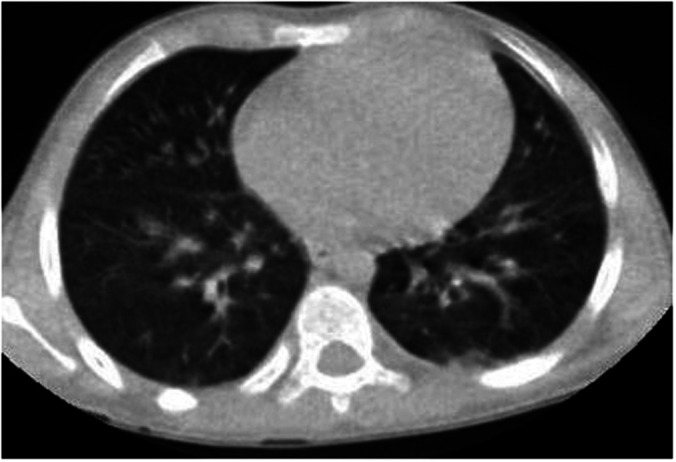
High-resolution computed tomography (HRCT) of a 9-year-old male patient with Kawasaki disease and COVID-19 pneumonia: Passive atelectatic changes on the right, ground-glass opacities near the pleural surface bilaterally and patchy ground-glass opacities in the middle lobe of the right lung. COVID-19: coronavirus disease 2019.
